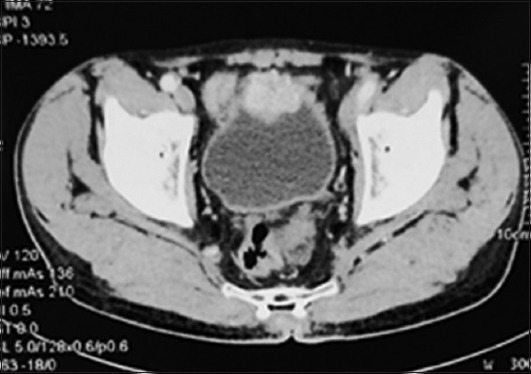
Contrast-enhanced CT exhibiting a hyperdense mass at the anterior wall of the urinary bladder. Abbreviations: CT: Computed tomography
